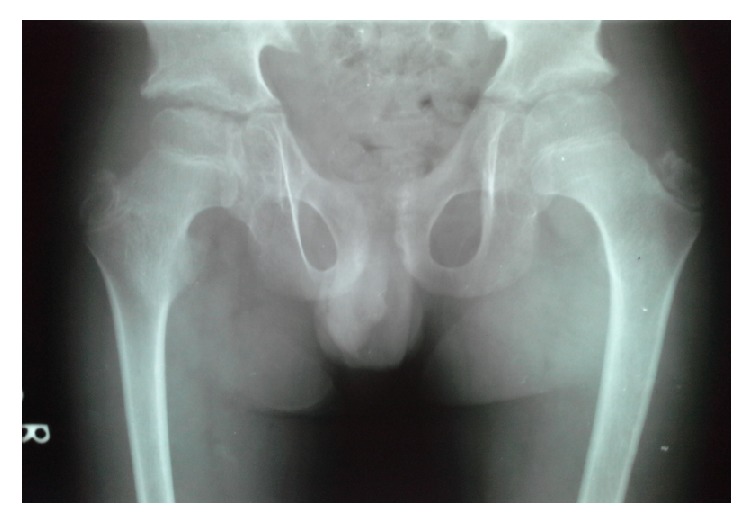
Anteroposterior X-ray of the pelvis showing flattened and enlarged epiphyses of the femoral heads.
